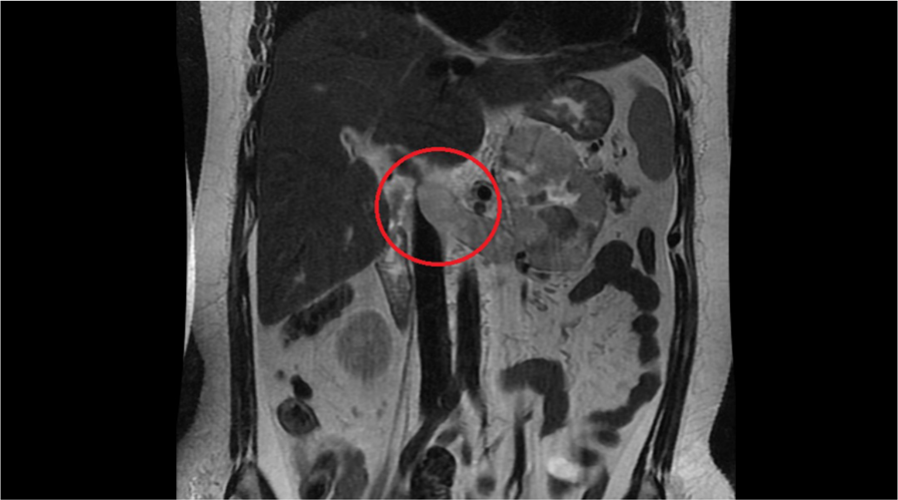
Abdominal magnetic resonance imaging. T1-weighted magnetic resonance image revealing a large (10.0 cm) tumor in the left adrenal gland extending into the inferior vena cava (circle) with the proximal position at the level of the hepatic vein
